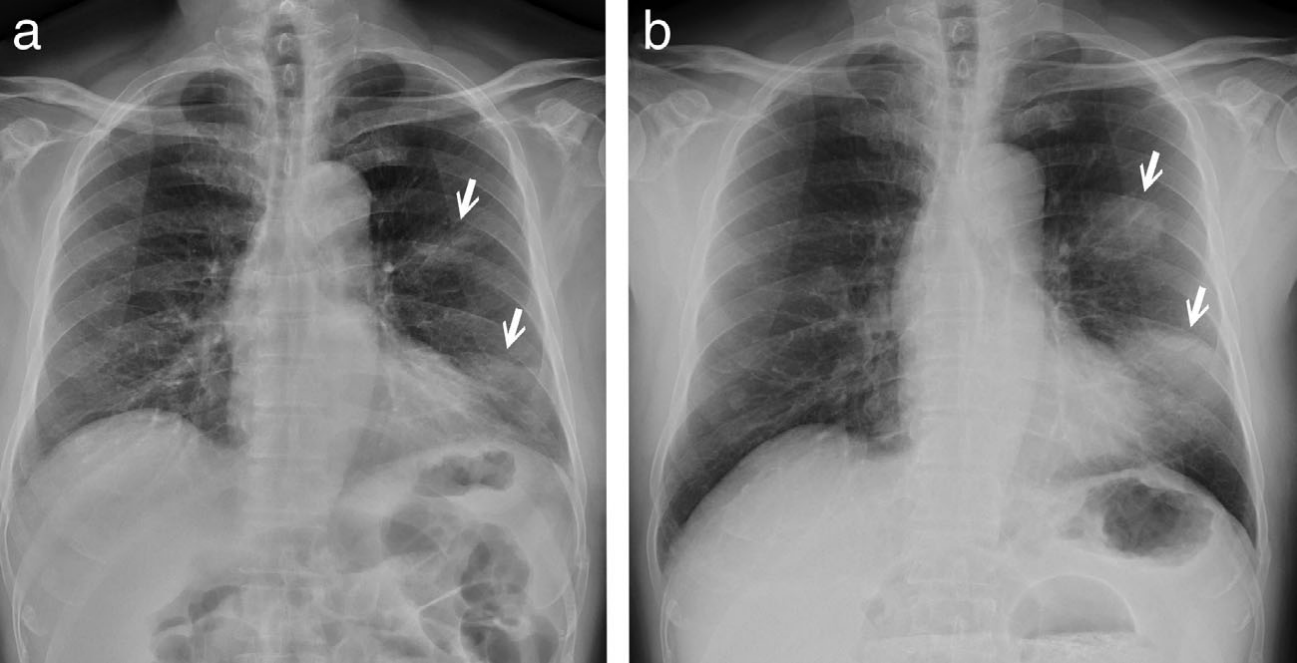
(a) Chest radiograph showed ill‐defined opacities in the left parahilar and left lower lung fields (arrows in a). (b) Follow‐up chest radiograph three months later revealed a rapidly developed well‐defined nodular and consolidated lesions in the left middle and lower lung fields (arrows in b).
